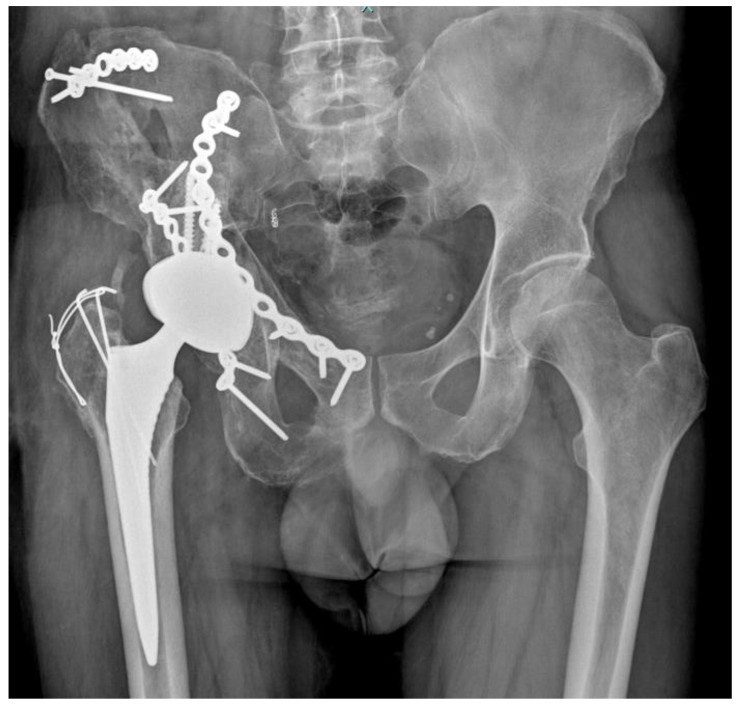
X-ray of the pelvis after the implant of total hip arthroplasty.
